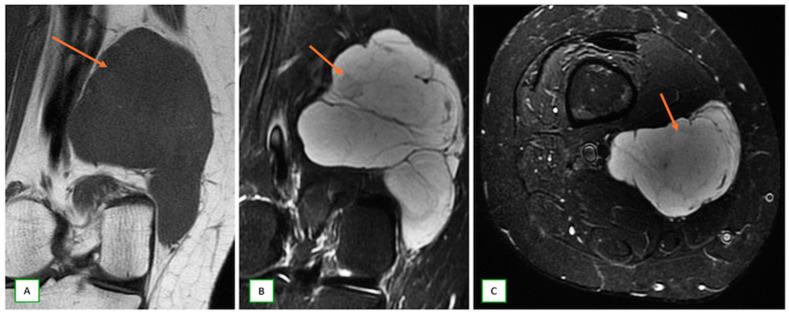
Myxoid nerve sheath tumor in a 59-year-old woman with a lump behind the knee. Coronal T1 (A), coronal (B), and axial (C) fat-saturated T2 images show a myxoid tumor (thin arrows) showing an extremely high signal on the T2-weighted images.
